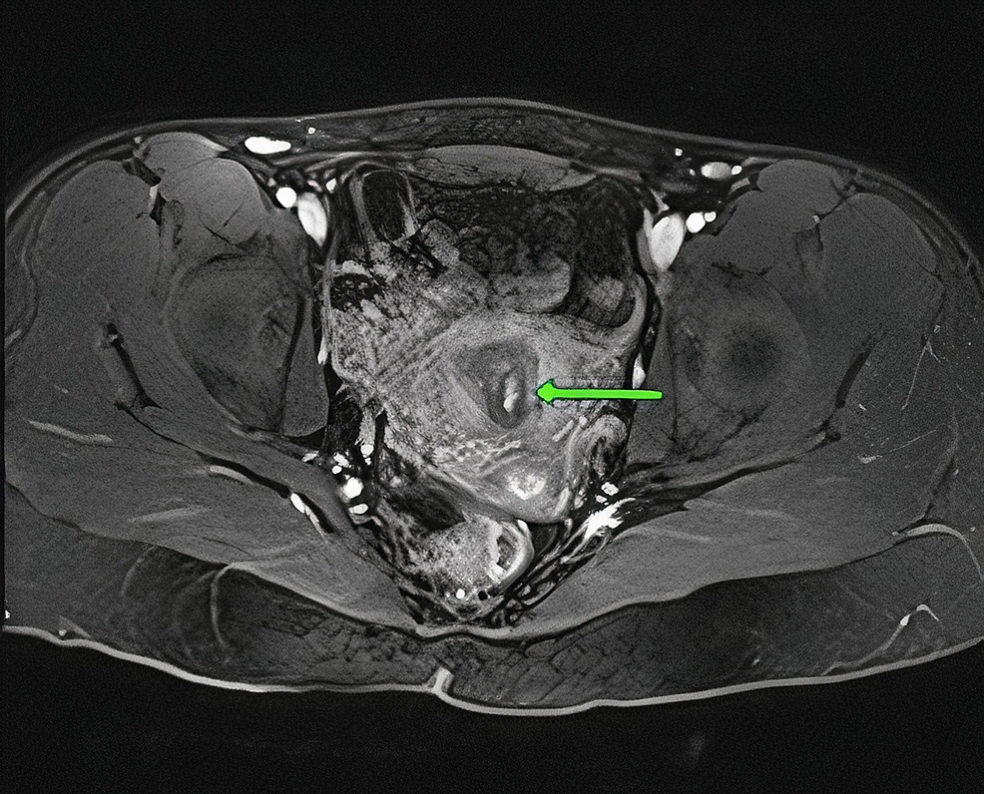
Pelvic MRI demonstrating arteriovenous malformation within the endometrial cavity.
